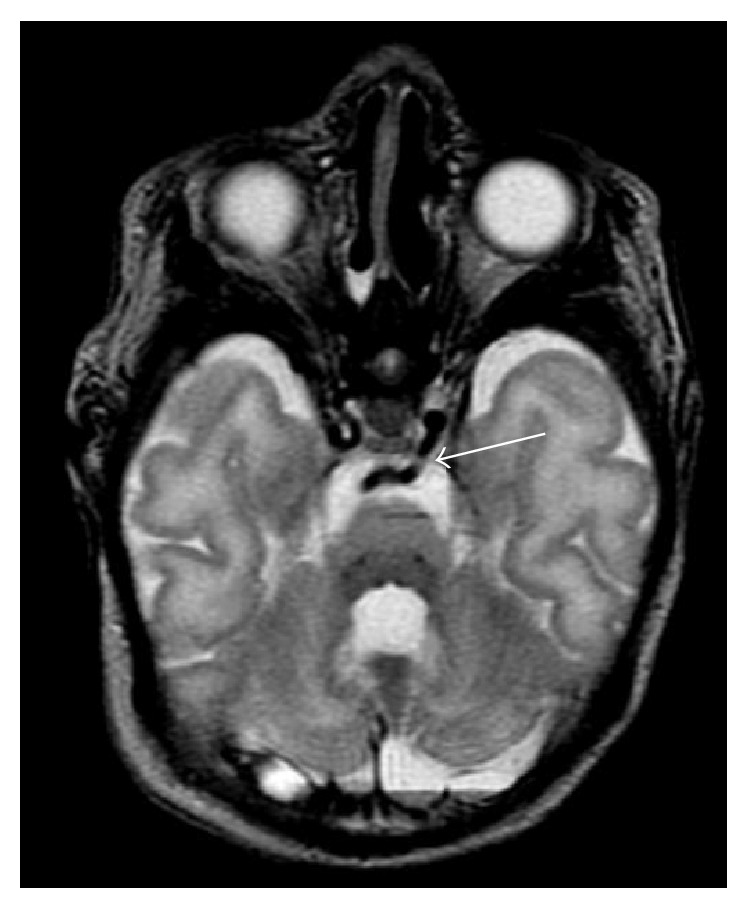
Axial T2-weighted MR image shows a persistent trigeminal artery (arrow) coursing from the cavernous left internal carotid artery posteriorly and medially to anastomose with the midbasilar artery.
